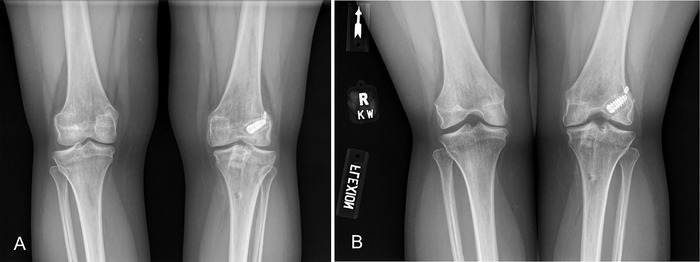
Six-week postoperative anteroposterior radiographs of both knees (A) in standing view and (B) at 45° flexion show appropriate positioning of the tunnels and hardware.
